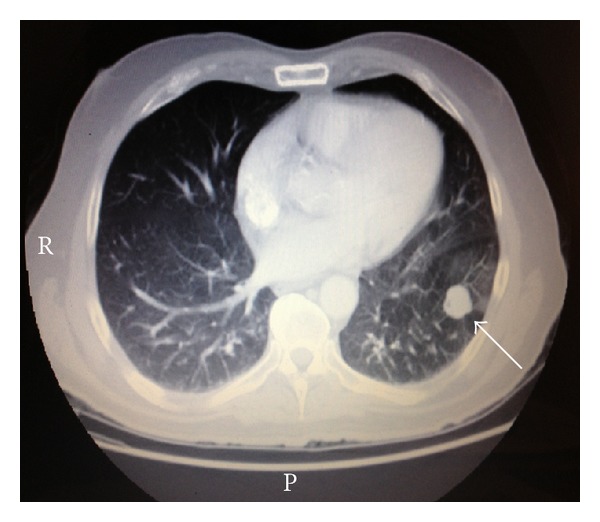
Staging CT scan showed a pulmonary nodule identified in the left lower lobe which is 1.8 × 1.8 cm in diameter.
